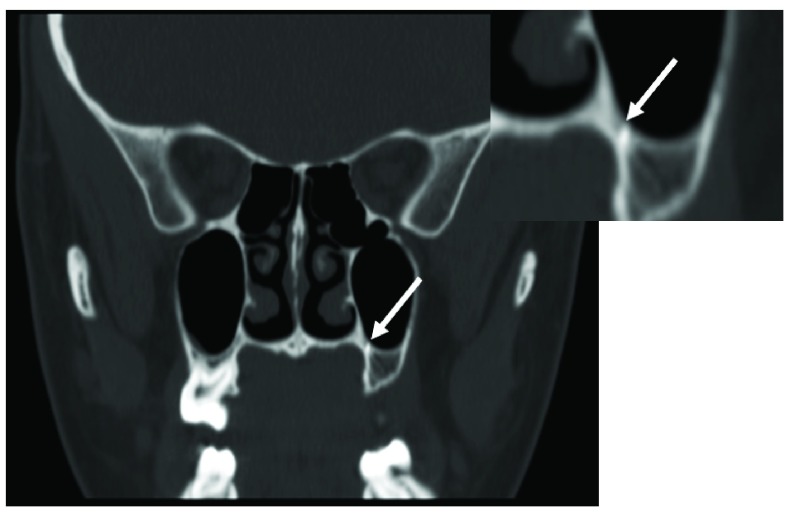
Repeat non-contrasted coronal CT scan. Retained gutta-percha (white arrow) noted in left maxillary sinus. Inset in the top right corner reveals a zoomed image of the gutta-percha.
